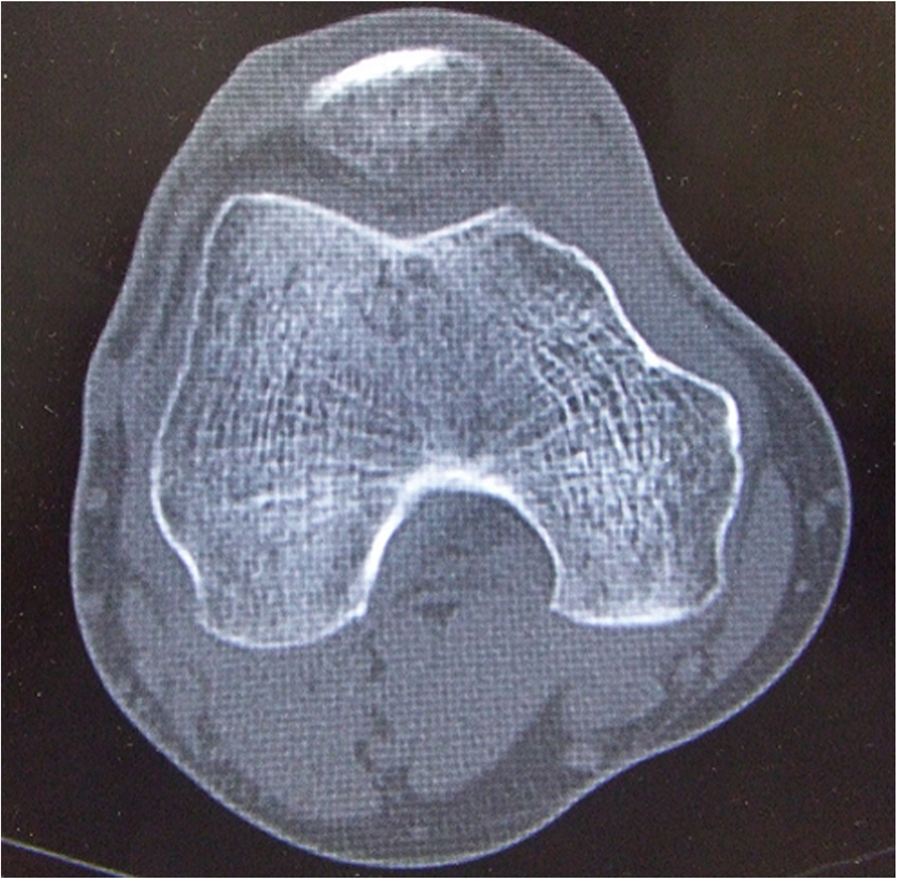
Axial computed tomographic image. The shape of the medial femoral condyle is partially concave
